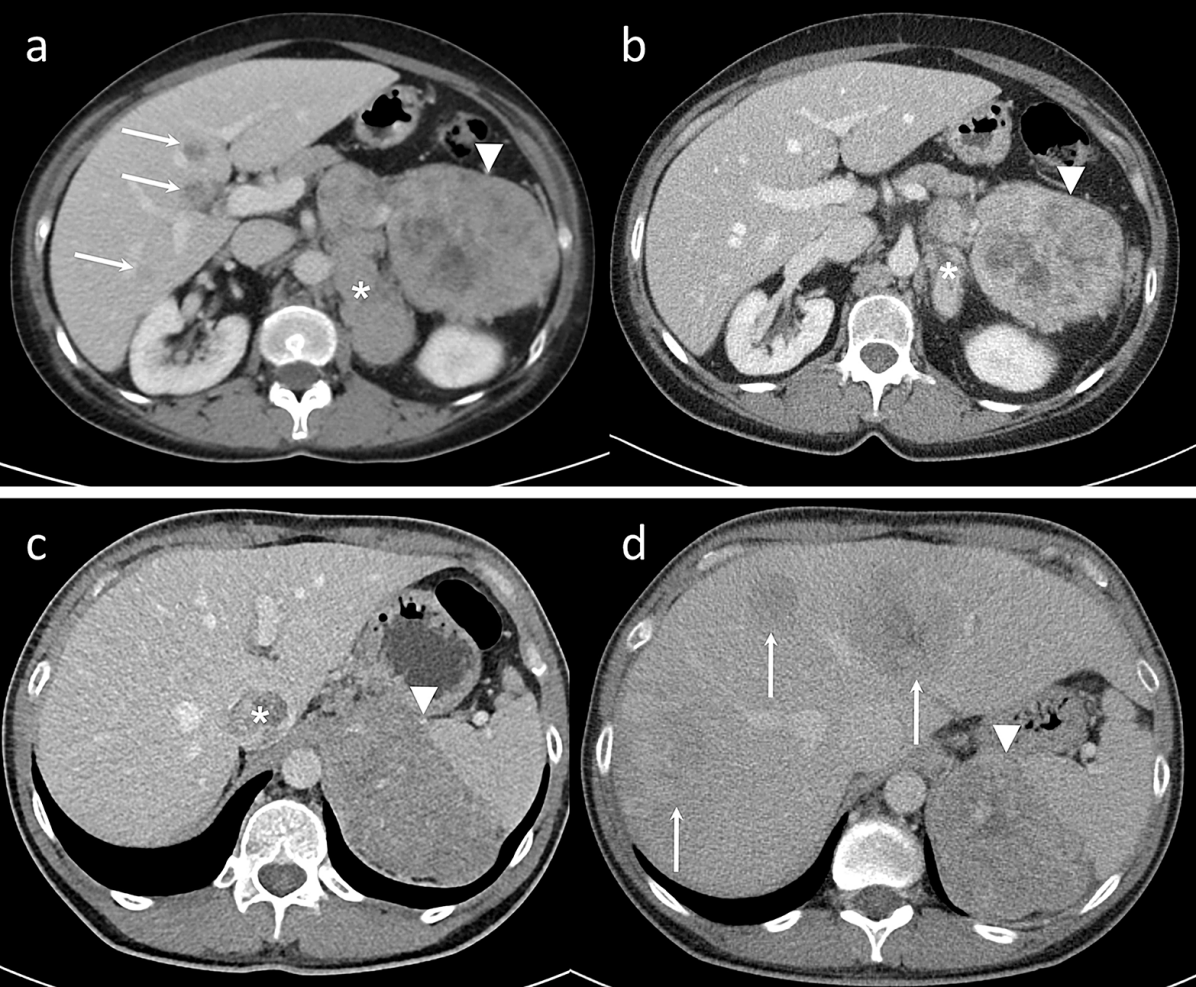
Contrast enhanced CT scans in venous phase EDP-M response in homogeneous and heterogeneous ACC. Responder patient: panel a) staging scan showing a large left adrenal lesion (arrowhead) with coarse and inhomogeneous texture (with high values of GLRLM_LRHGLE and Kurtosis), large loco-regional nodal metastases (asterisk) and liver metastases (arrows); panel b) restaging scan after EDP-M therapy with a reduction of the adrenal lesion (arrowhead) and of the locoregional metastases (asterisk) and disappearance of the liver metastases. Non-responder patient: panel c) staging scan showing a large left adrenal lesion (arrowhead) with fine and homogeneous texture (with low values of GLRLM_LRHGLE and Kurtosis) and a thrombus in the inferior vena cava (asterisk); panel d) restaging scan after EDP-M therapy with a slight reduction of the adrenal lesion (arrowhead) and the disappearance of the inferior vena cava thrombus but with the comparison of multiple liver metastases (arrows)
